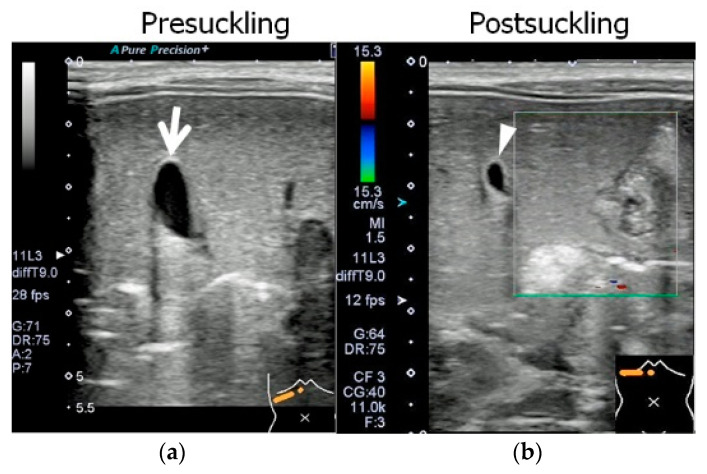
Abdominal ultrasonographic findings in Case 1 before and after feeding; Aplio 300 (Cannon Medical Systems), linear probe, B-mode frequency: 9.0 MHz, pulse repetition frequency: 12 fps. Images are presented in the right upper abdominal region, on the same scale as in Figure 1. (a) The gallbladder was examined at the same time as in Figure 1. The gallbladder lumen (arrow) was open. The measurements of the gallbladder were a long diameter of 18.0 mm, short diameter of 7.2 mm, and lumenal cross-sectional area of 0.53 cm^2^. The gallbladder wall, which was 1.0 mm thick and exhibited a two-layered appearance, was normal in structure. (b) After 30 min, another ultrasonographic examination was performed after the completion of 20 min autonomous feeding. Because the gallbladder had contracted and become twisted in structure, it was impossible to view the region from the gallbladder base (arrowhead) to the cholecystic duct on the same screen. The measurements of the gallbladder were a long diameter of 10.9 mm, short diameter of 6.0 mm, and lumenal cross-sectional area of 0.27 cm^2^.
